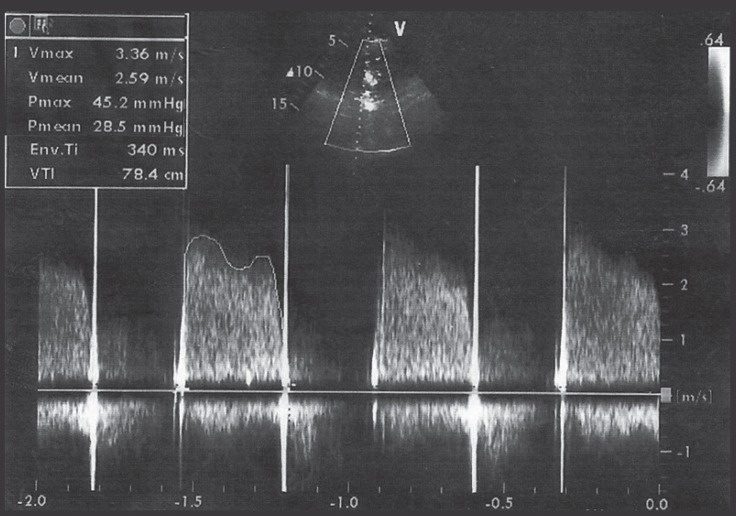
Transthoracic echocardiography reveals the obstruction of the prosthetic mitral valve with a mean diastolic mitral transvalvular gradient of 23 mm Hg and a peak velocity of 3.36 m/s.
